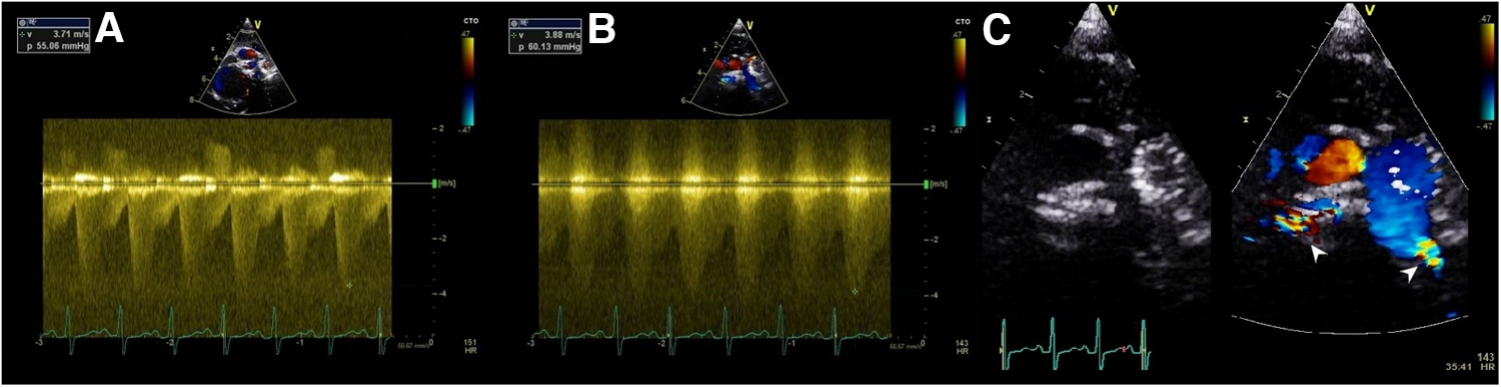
Ultrasound Doppler tracing showing a systolic-diastolic flow profile of effective PFRs in LPA (A) and RPA (B) of patient no.5. 2D and color-Doppler ultrasound short-axis view of PFRs in both PA branches (white pointed arrows) (C).
